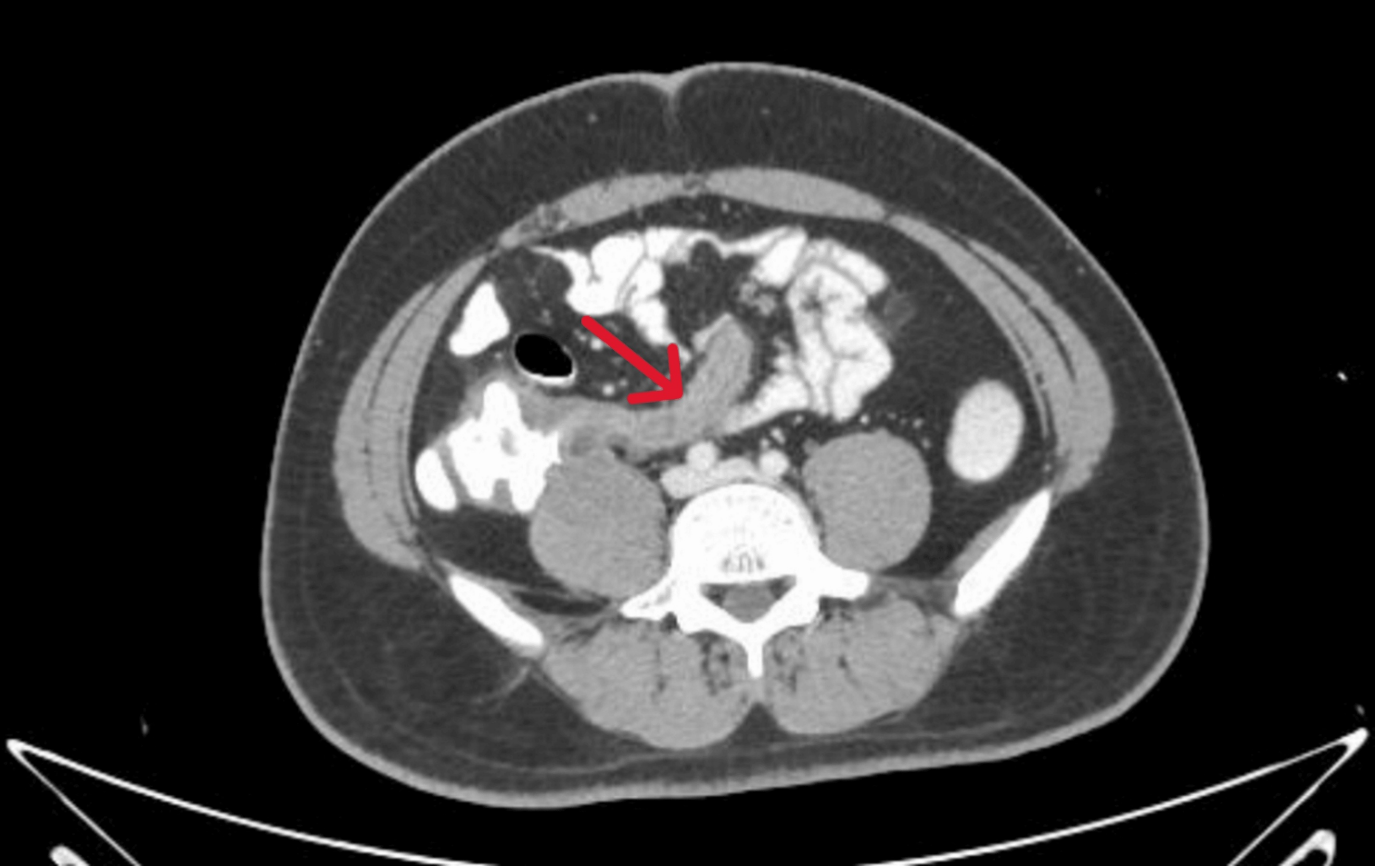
Contrast-Enhanced CT Depicting Acute Appendicitis with Periappendiceal Abscess (Case 1) Axial contrast-enhanced CT (CECT) of the abdomen shows a dilated and inflamed appendix (red arrow) with wall enhancement and surrounding fat stranding. A thick-walled hypodense collection adjacent to the appendix is consistent with a periappendiceal abscess. The caecal wall appears thickened, with associated mesenteric lymphadenopathy, indicating complicated appendicitis.
